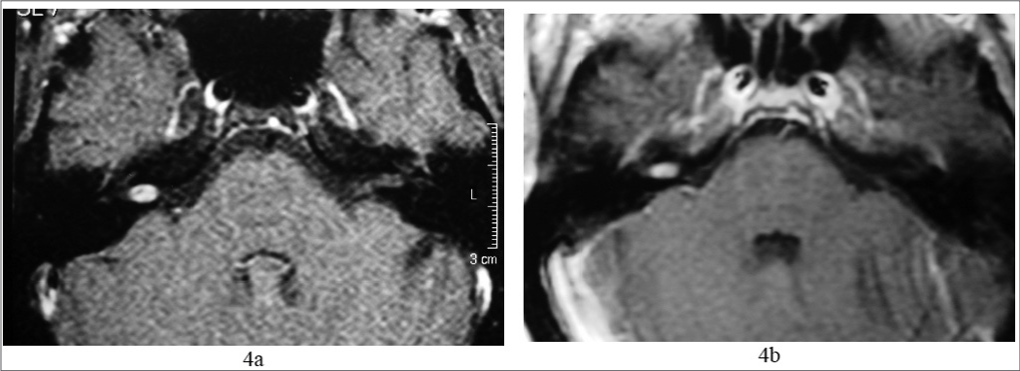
4 a) MRI with gadolinium at the time of diagnosis; 4b) after two years.
